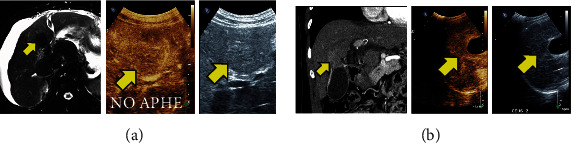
(a) T2-weighted MRI of the liver (left panel) demonstrates a T2 hypointense lesion (arrow) in the central liver. Although a discrete nodule was visualized on grayscale US (right panel), no arterial phase hyperenhancement (APHE) was seen on CEUS (middle panel) consistent with a cirrhotic-associated regenerative nodule (CEUS LI-RADS 3). (b) Coronal contrast-enhanced CT image (left panel) demonstrating a hyperdense region adjacent to the gallbladder (arrow). This region enhances similar to background parenchyma on CEUS (middle panel), and no discrete lesion is visualized on greyscale US (right panel) consistent with a pseudolesion.
